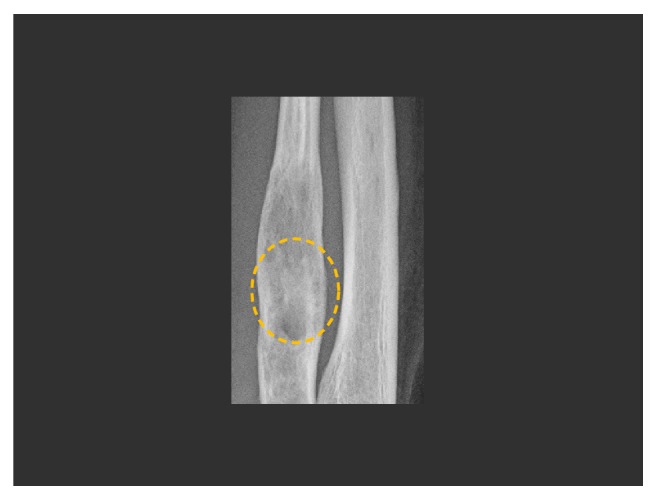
A follow-up radiograph 8 weeks after surgery demonstrates partial filling in of the lucent lesion with healing bone (dashed circle) following curettage.
